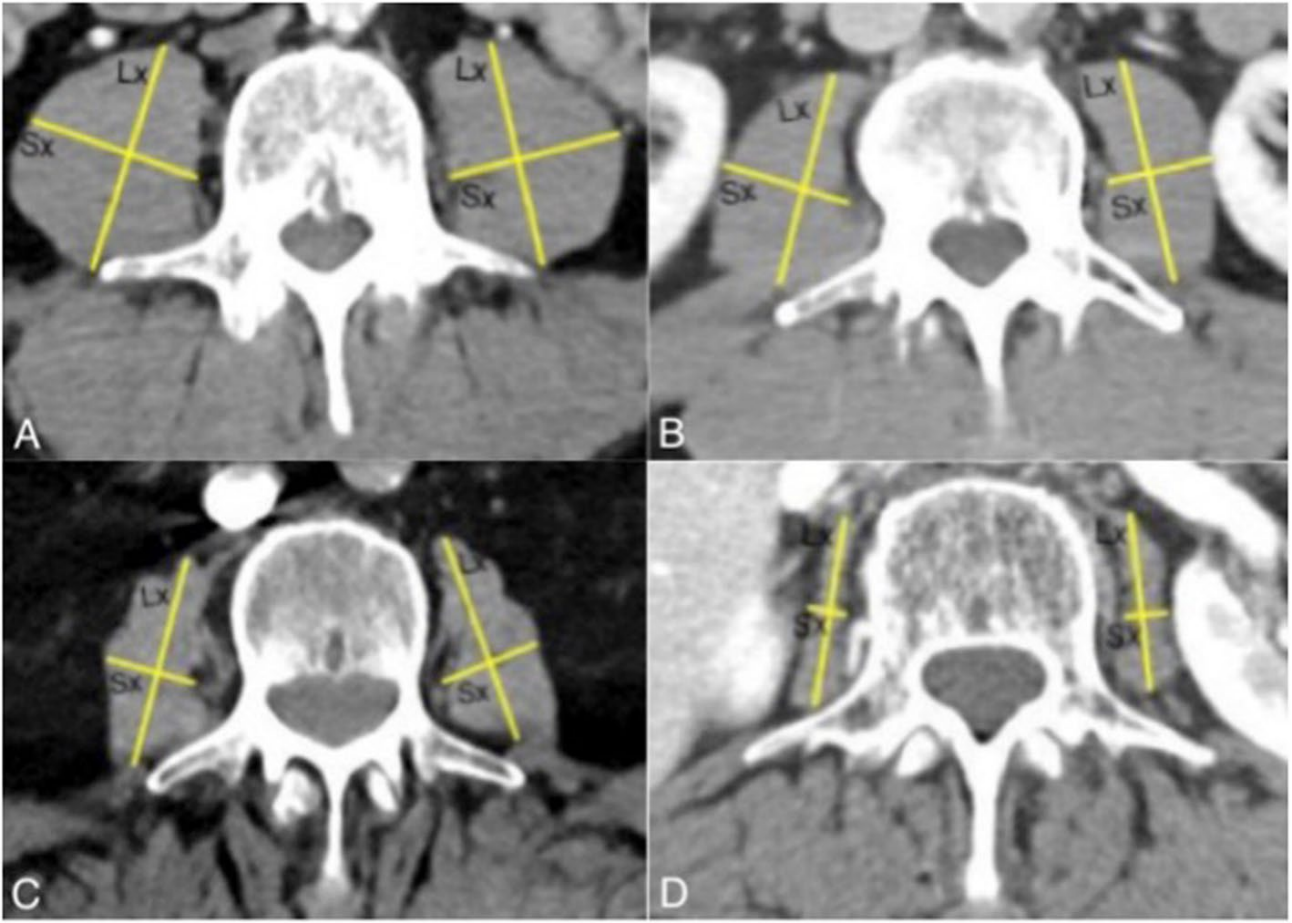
Measuring MPM in CT-images of axial views at the level of the L3 vertebra of a soft tissue window (Lx:Long axis, Sx: short axis; A = stadium 0, B = stadium 1, C = stadium 2, D = stadium 3)
